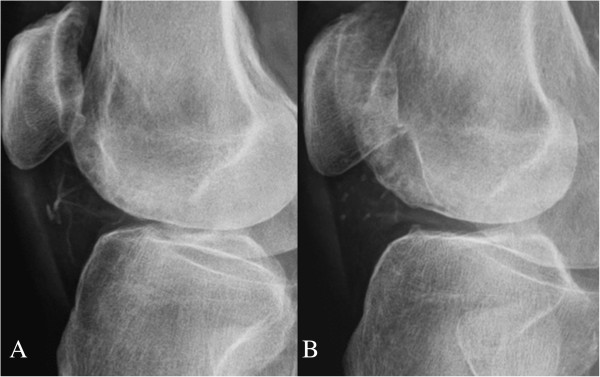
Plain radiographs of the right knee. (A) Before specific treatment, the joint space exhibited obvious small vessel calcification. (B) After 11 months, the small vessel calcification showed substantial regression.
